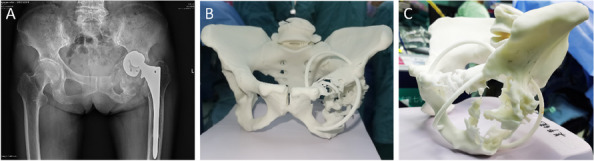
A, B and C Pictures showed preoperative pelvic X-ray and 3D reconstruction of one typical case
